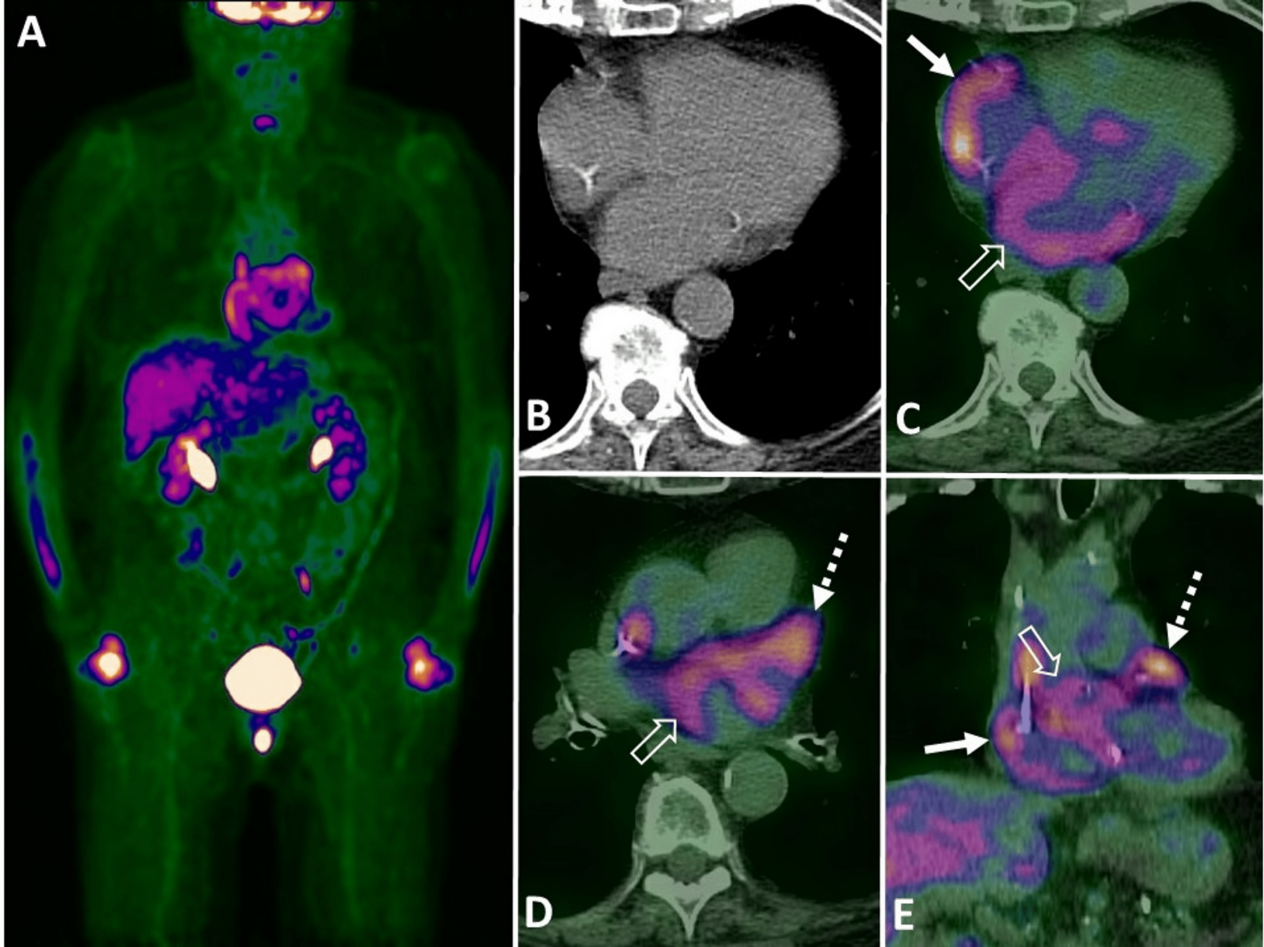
Surveillance fluorine 18 (18F) fluorodeoxyglucose (FDG) PET/CT images (A) maximum intensity projection, (B) unenhanced axial CT image, (C-D) transaxial fused PET/CT images, and (E) coronal reformatted fused PET/CT image demonstrates abnormal focus of increased FDG uptake in the right atrium (solid white arrow in C and E), left atrium (open white arrow in C-D) and left atrial appendage (dashed white arrow in D-E) without structural abnormality in unenhanced CT.
